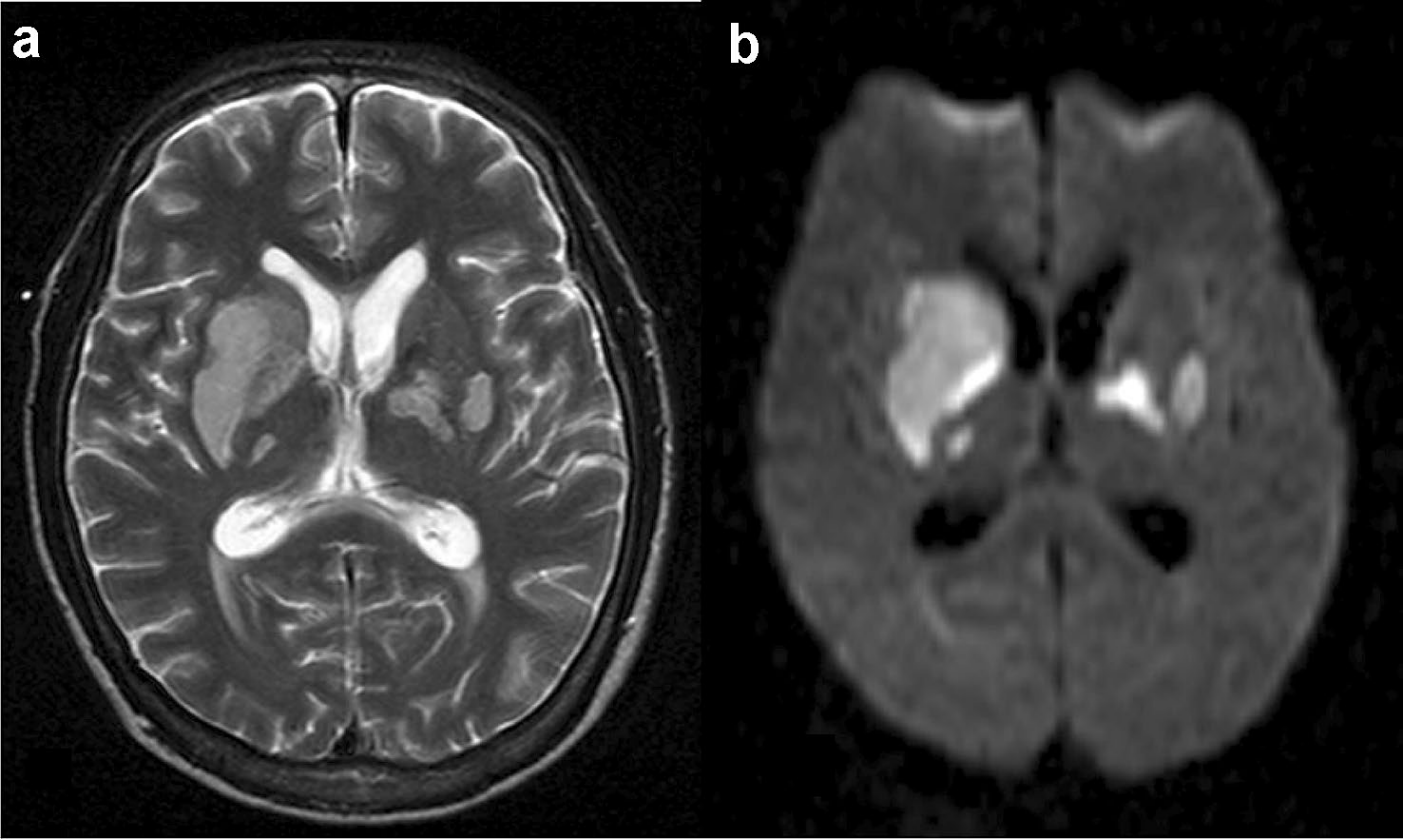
Acute infarction caused by tuberculosis meningitis in a patient with HIV infection. Hyperintensity on T2-weighted image (a) is shown in the bilateral basal ganglia with slight swelling. The lesion shows hyperintensity on diffusion-weighted image corresponding to acute infarction (b). Enlargement of the bilateral lateral ventricle revealing hydrocephalus was also noted
